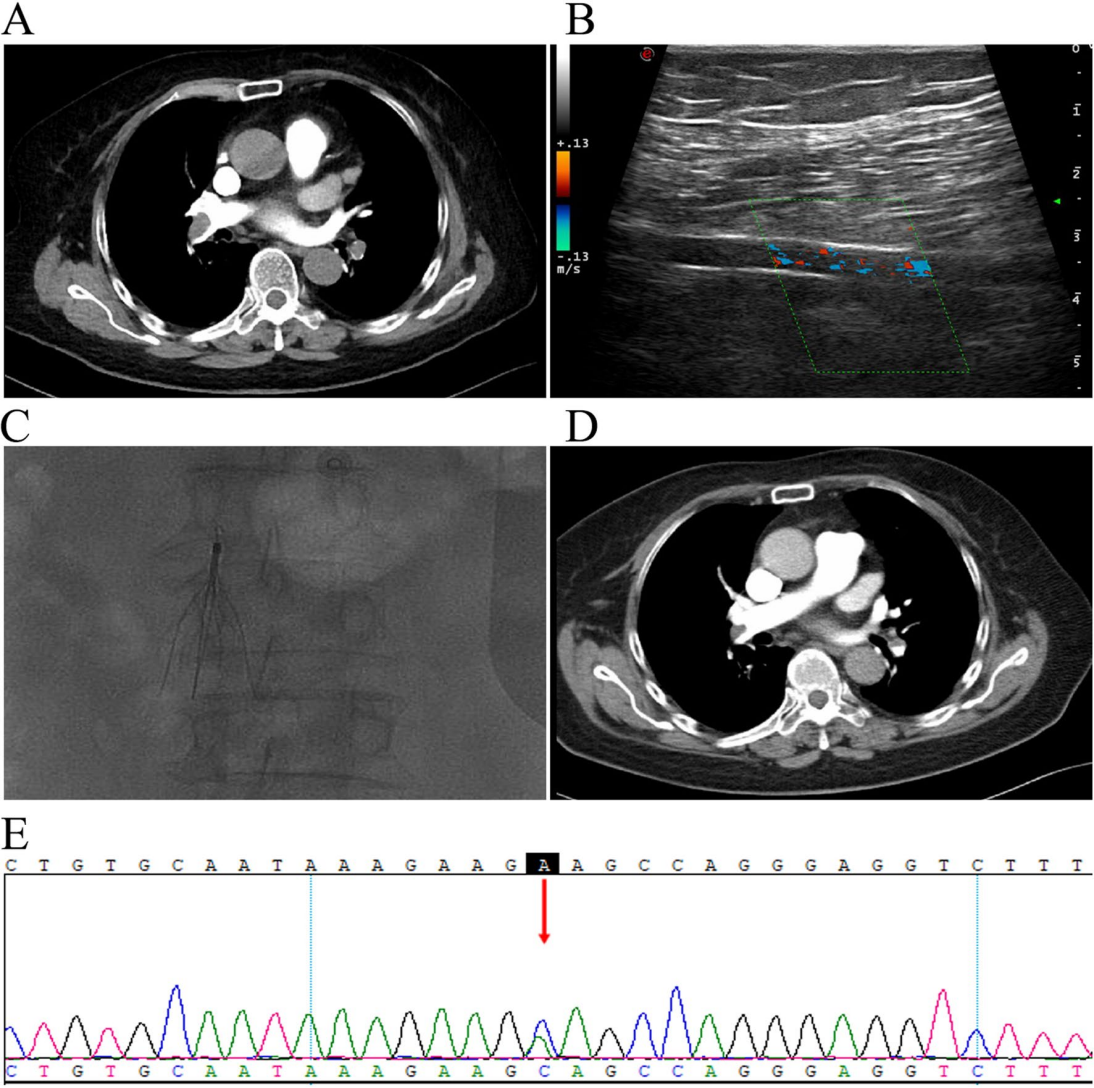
A, B Computed Tomography Pulmonary Angiography and lower extremity venous ultrasound at initial hospitalization: suggestive of a large filling defect in the right pulmonary artery trunk, consistent with pulmonary embolism. A hypoechoic mass with no blood flow signal was found in the left popliteal vein, suggestive of lower extremity deep vein thrombosis. C Placement of filter in inferior vena cava. D After 2 weeks of anticoagulant therapy, Computed Tomography Pulmonary Angiography showed a decrease in the size of the main pulmonary artery thrombus. E Whole exome sequencing revealed a heterozygous NM000313.3: C200A>C mutation in the PROS1 gene in the patient. Arrows: Sequencing double peaks indicate an A to C mutation
